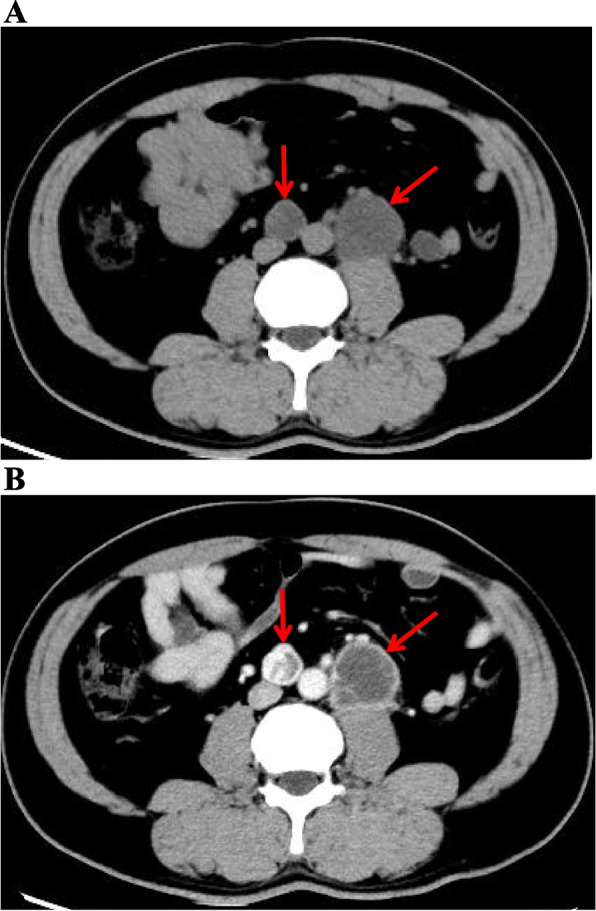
A and B Abdominal computer tomography plain and enhanced scan. Masses and mixed low-density shadow on both sides of the abdominal aorta, and marked with a red arrow. The larger mass was located on the left side and measured 4.01×3.94cm; it was visible thin walls and partitions; the CT value was approximately 14HU; and the enhanced scan revealed annular enhancement. CT: computer tomography
